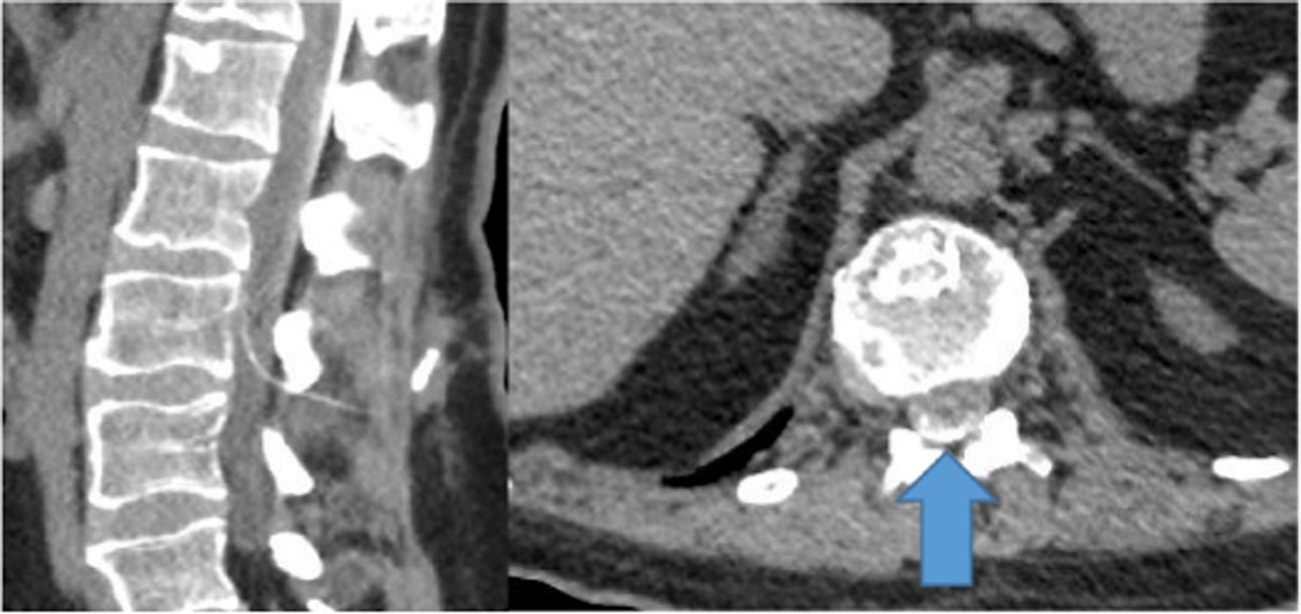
Intrathecal contrast after catheter access port contrast injection on CT (blue arrow)
